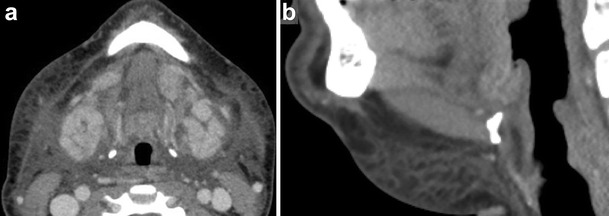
Ludwig angina. a Axial and b sagittal contrast-enhanced CT images show diffuse inflammatory changes with bands of fluid within the submandibular and sublingual spaces. These changes extend posteriorly into bilateral parapharyngeal spaces, pharyngeal mucosa, and inferiorly along the anterior neck (b)
